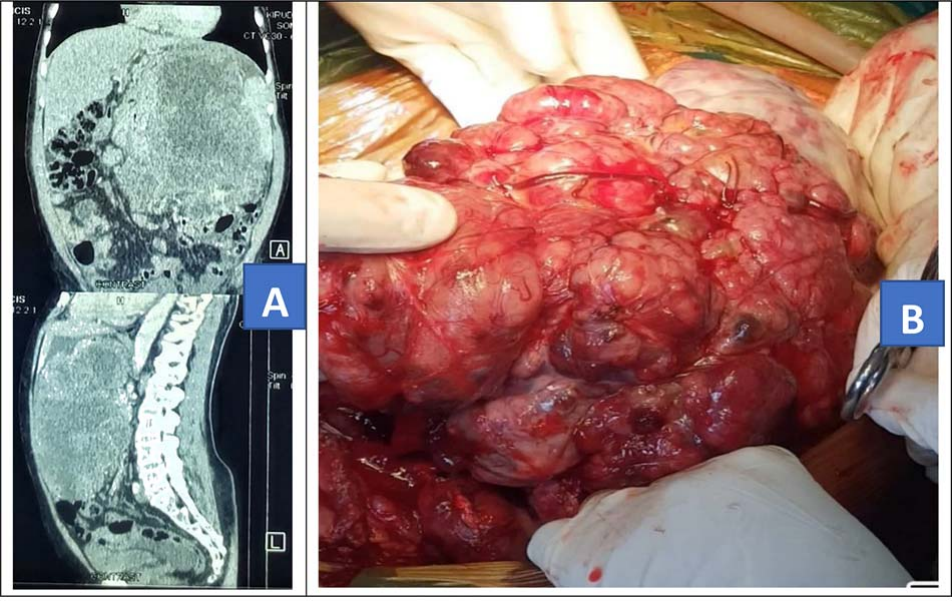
Contrasted abdominal CT scan (A) and intra-operative picture (B) for Patient 1.
